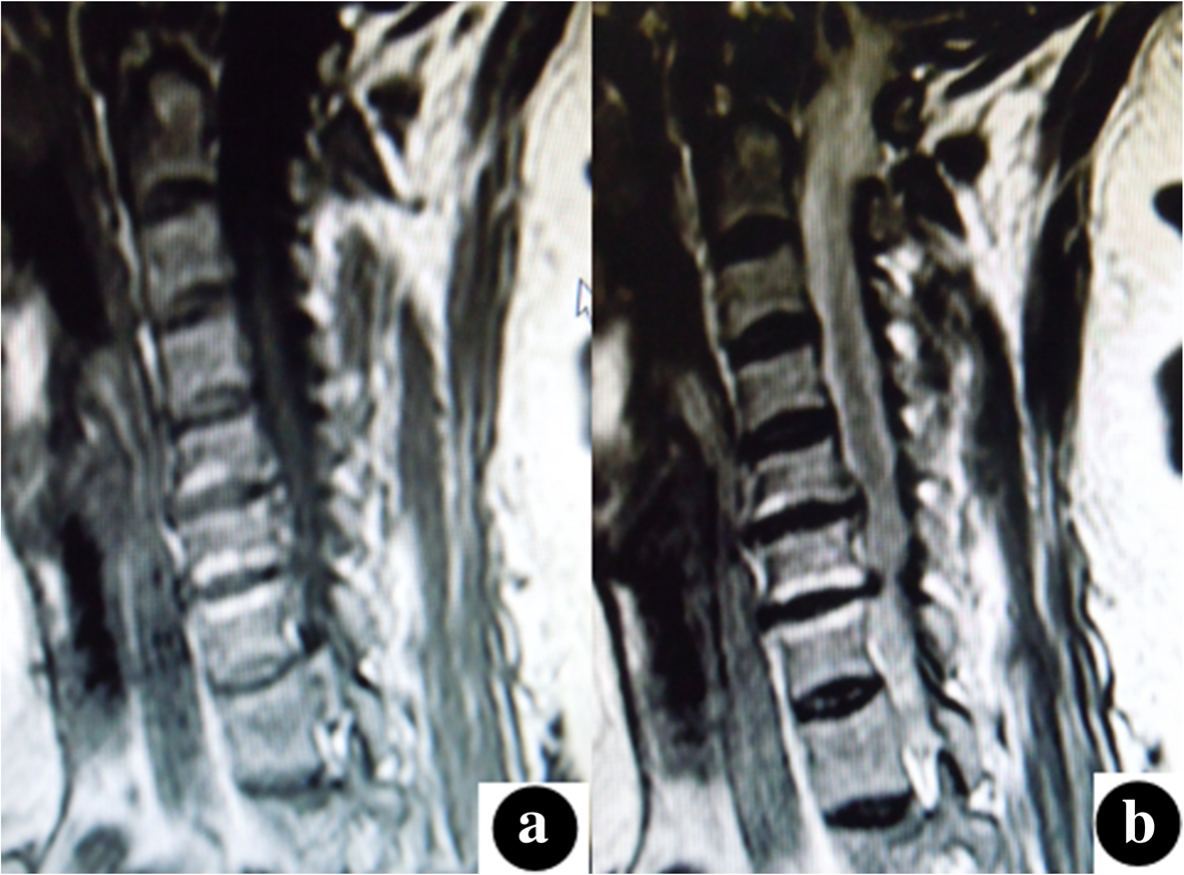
Modic type II changes. High signal intensity on T1-weighted (a) and T2-weighted (b) images are noted on both endplates at the C6–7 level in the cervical spine
